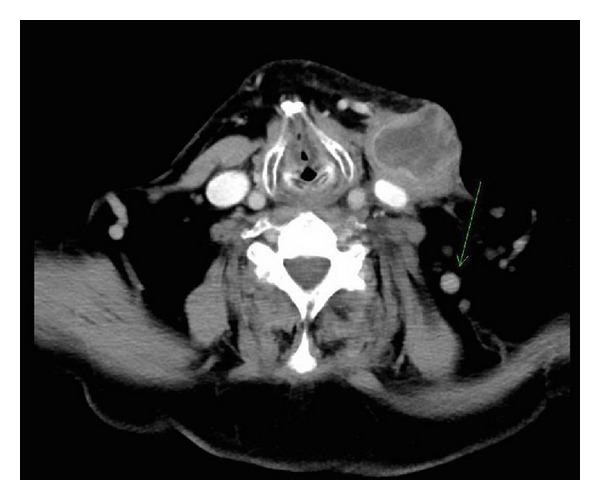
CT scan 6 months post-op, showing haemorrhagic node and level V lymphadenopathy (arrow).
